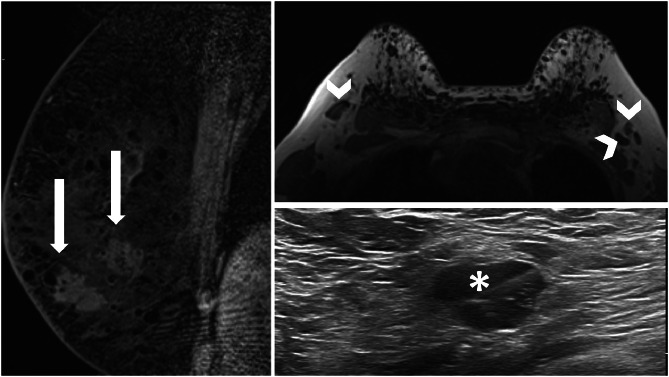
Left image – MRI right breast (postgadolinium T1 sagittal SPAIR sequence) demonstrated 2 hyperenhancing right lesions at 6 o'clock 5cm from the nipple (29mm lesion more superficial (left arrow) and 24mm deeper lesion) (right arrow), consistent with breast malignancies (MRI-guided biopsy proven). Top right image – MRI Breast (T1 axial sequence) demonstrated several enlarged (cortex > 3mm) bilateral axillary lymph nodes (arrowheads). Bottom right image – Ultrasound-guided biopsy of enlarged right axillary lymph node (asterisk) demonstrated metastatic breast malignancy.
